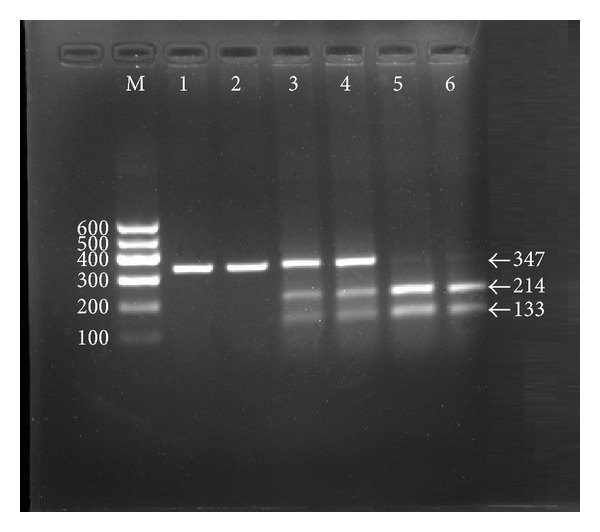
Genotyping of the *KLF14* rs4731702 SNP. Lane M, 100 bp marker ladder; lanes 1 and 2, TT genotype (347 bp); lanes 3 and 4, CT genotype (347-, 214-, and 133-bp); lanes 5 and 6, CC genotype (214- and 133-bp).
